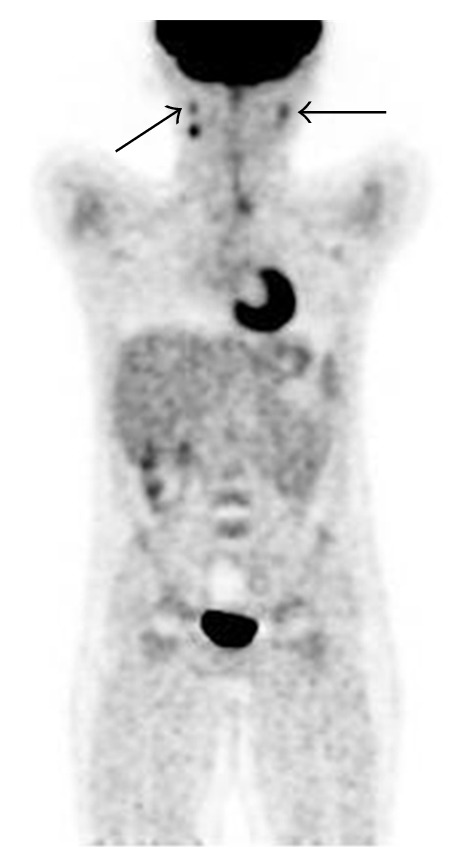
PET-CT of whole body shows two right and one left small hypermetabolic nodal lesions in the upper jugulodigastric area (black arrows). They are reactive in nature, otherwise negative scan.
